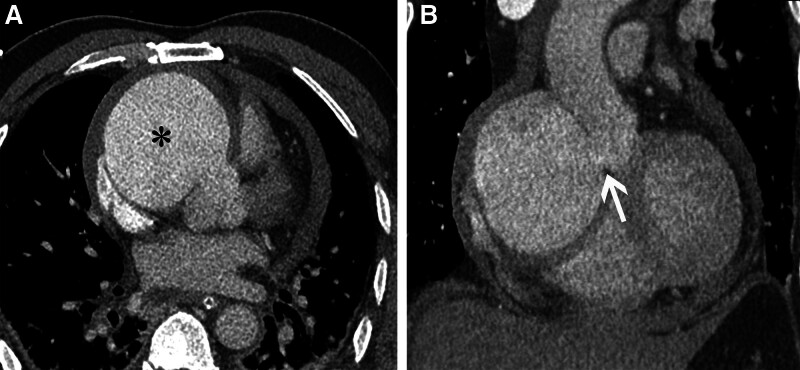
Preoperative chest CTA images of the patient. (A) The axial section shows a spherical high-density contrast-filled image (black asterisk). (B) Oblique coronal section revealing a rupture measuring 1.6 × 2.0 cm at the right coronary sinus (white arrow). CTA = computed tomography angiography.
